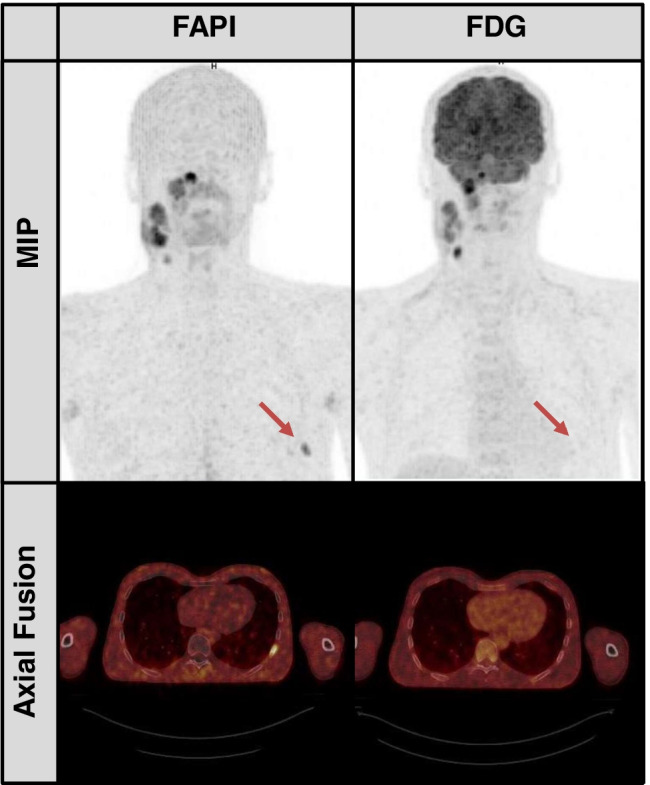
False-positive uptake in FAP-scans in a patient’s left rib. Maximum-intensity projections and axial fusion images of a 37-year-old male with nasopharyngeal cancer prior to radiation therapy. The patient had FAP uptake in the left 9th rib rated as benign (red arrow). Neither the FDG scan nor conventional imaging was suggestive of bone metastasis.
